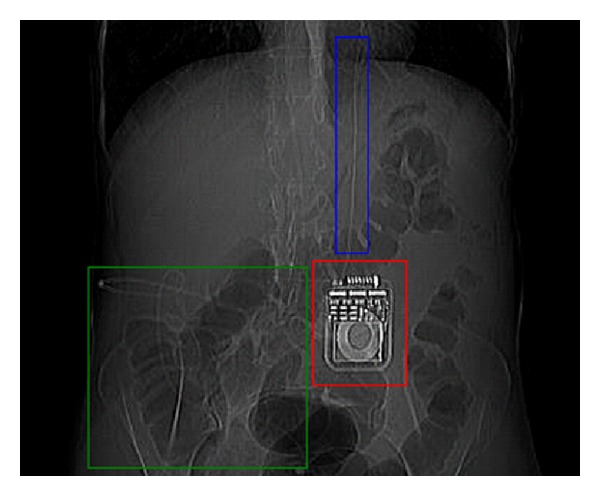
A preinsufflation “scout” view from the patient's CT colonography. Red box: the occipital nerve stimulator generator; blue box: wire from occipital nerve stimulator generator; and green box: course of lumboperitoneal shunt.
